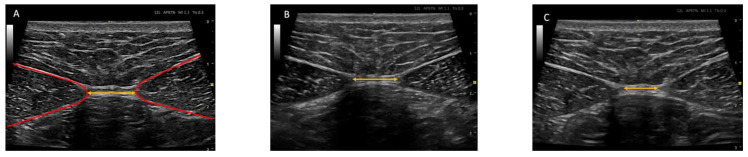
Ultrasound images of the rectus abdominis distance (IRD). (A) Right and left abdominal rectus abdominis (areas between the red lines) and intermediate fascia corresponding to the IRD (represented by the yellow line). (B) Ultrasound image of the IRD at rest. (C) Ultrasound image of the IRD during muscle activity.
